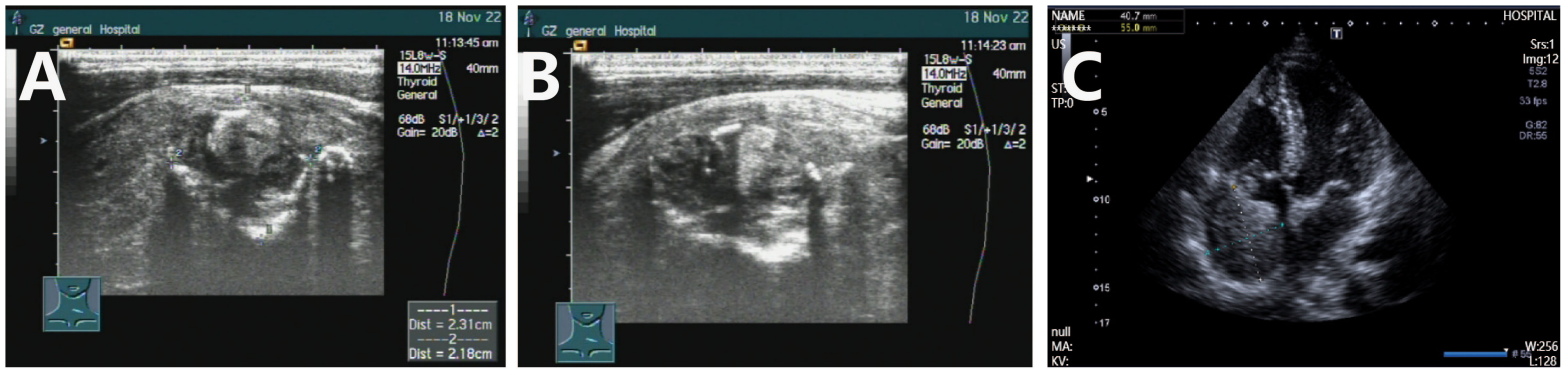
Color ultrasound examination of thyroblastoma. (A, B) Ultrasound results obtained on November 18 indicated an increased volume of both thyroid lobes, with heterogeneous internal echoes and multiple solid hypoechoic nodules, accompanied by calcification. (C) On May 20, 2024, cardiac ultrasound revealed the presence of a slightly hyperechoic mass within the right atrium, accompanied by enlargement of the right atrium and right ventricle.
